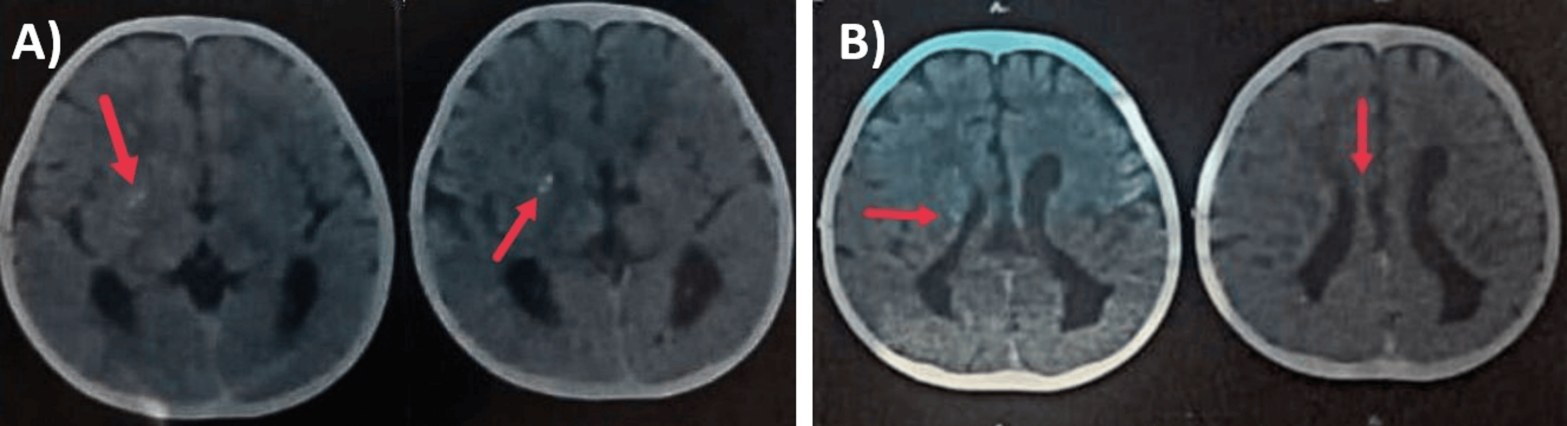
Computed Tomography (CT) of Brain A) CT Brain showing calcifications over basal ganglia; B) CT Brain showing calcifications in periventricular white matter
